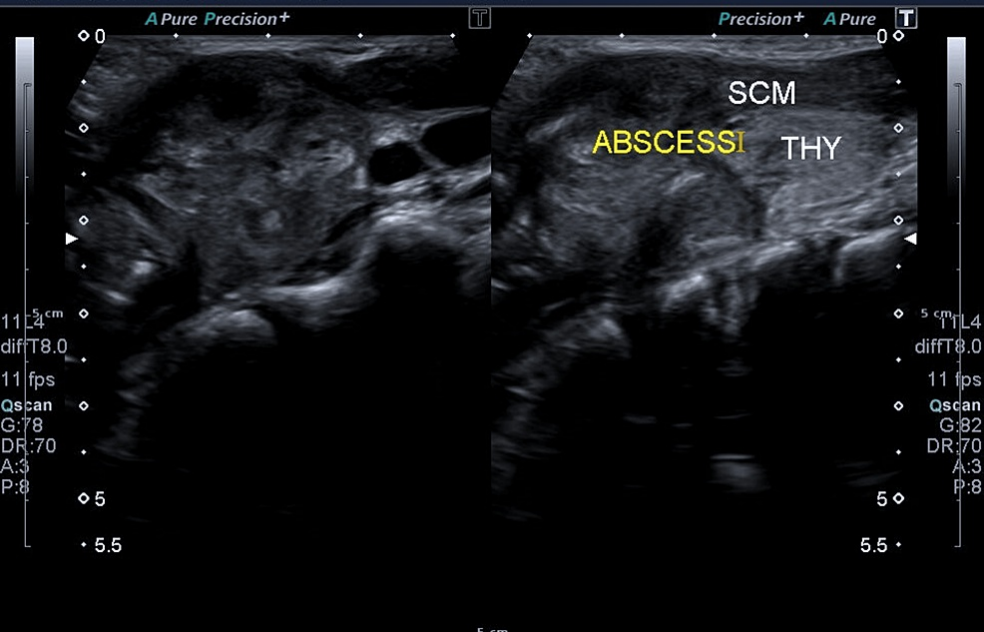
This ultrasonography image of the neck shows a large, vascularized area in the left thyroid lobe, potentially indicating an abscess, along with enlarged cervical lymph nodes nearby. SCM: sternocleidomastoid, THY: thyroid
